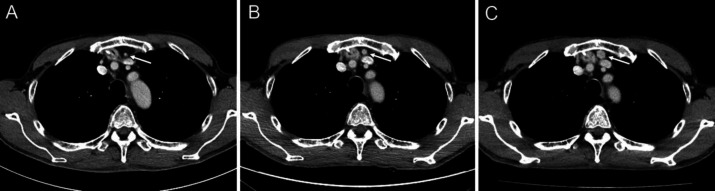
Serial postoperative contrast-enhanced CT of the chest at 3 months (A), a year and 3 months (B), and 2 years and 3 months (C) postoperatively. A small nodule is identified near the reconstructed gastric tube, showing gradual enlargement (arrows)
